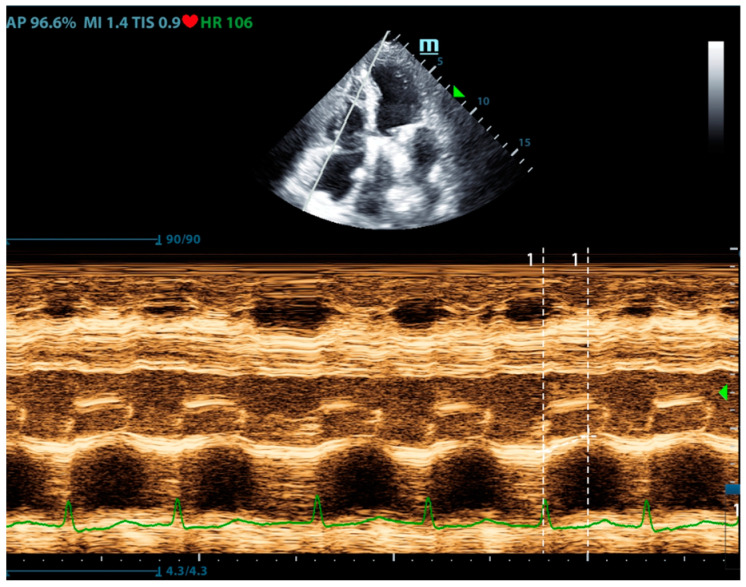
The ultrasound measurement of TAPSE.
